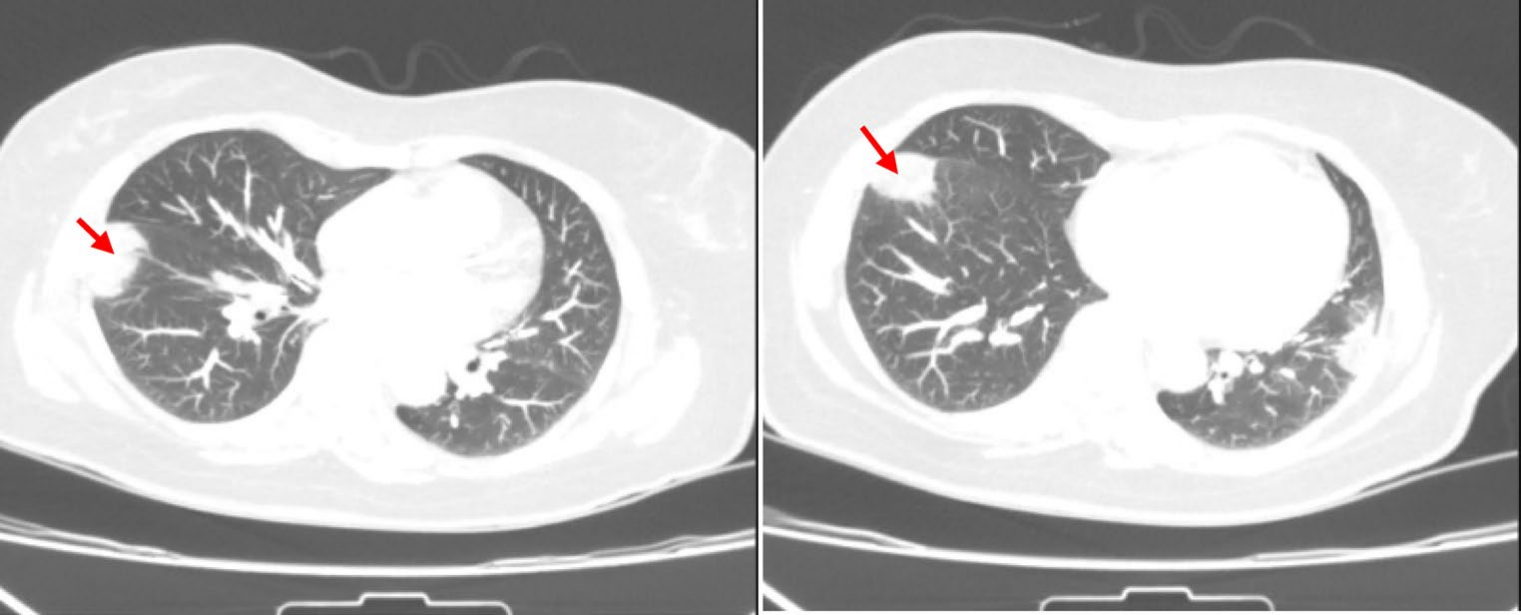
Non-contrast CT showing right lower lobe subpleural wedge-shaped opacity (red arrow) consistent with infarction
